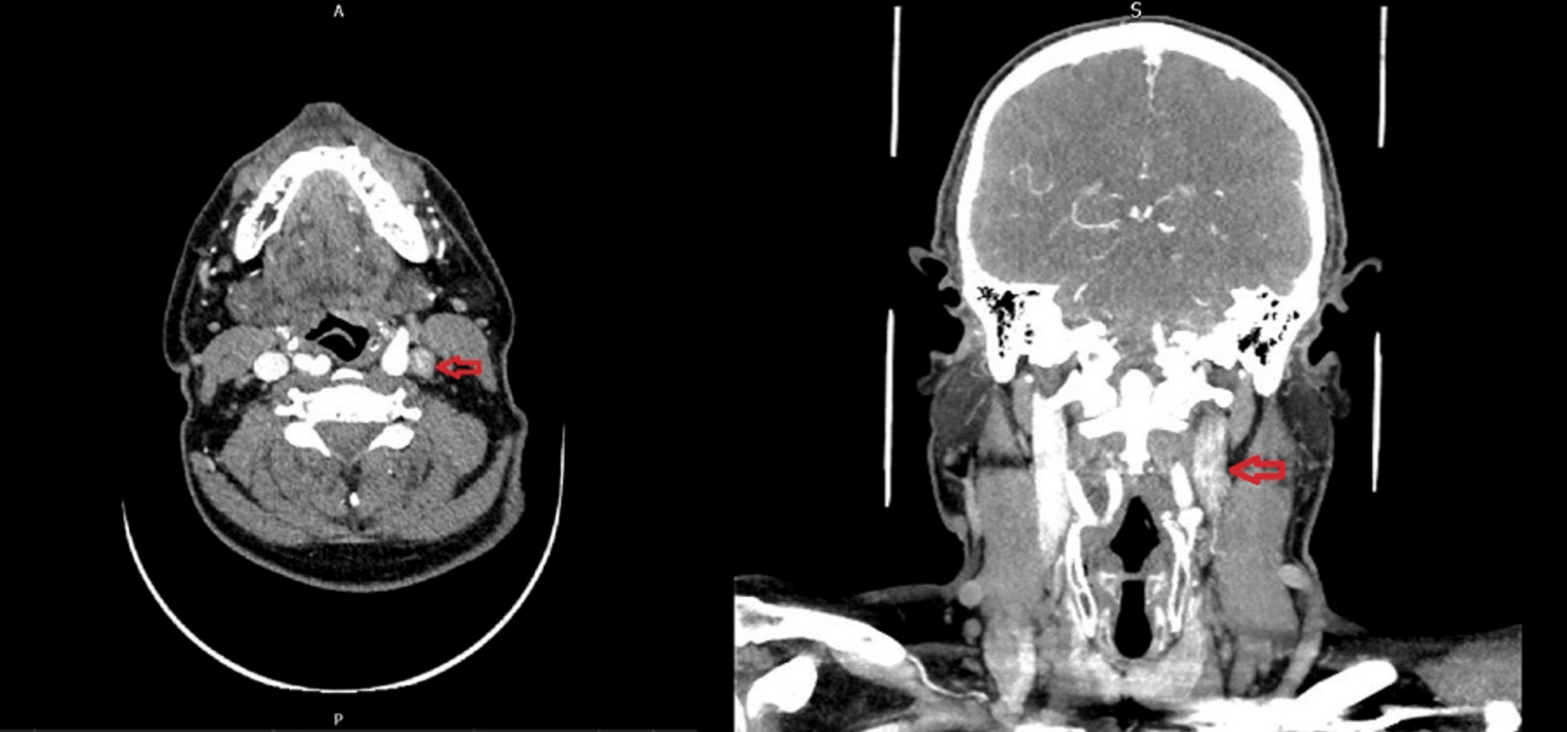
CT angiogram. Axial and coronal CT images demonstrating circumferential narrowing of the left internal carotid artery (ICA).
